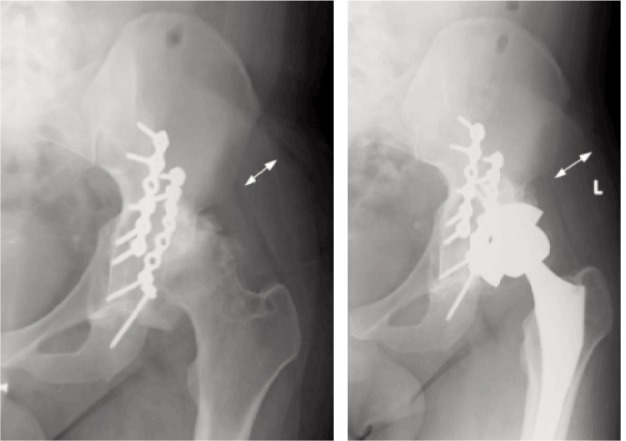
Radiograph at 2 years showing degenerative hip changes and increase in gluteus medius girth, prior to total hip arthroplasty.
